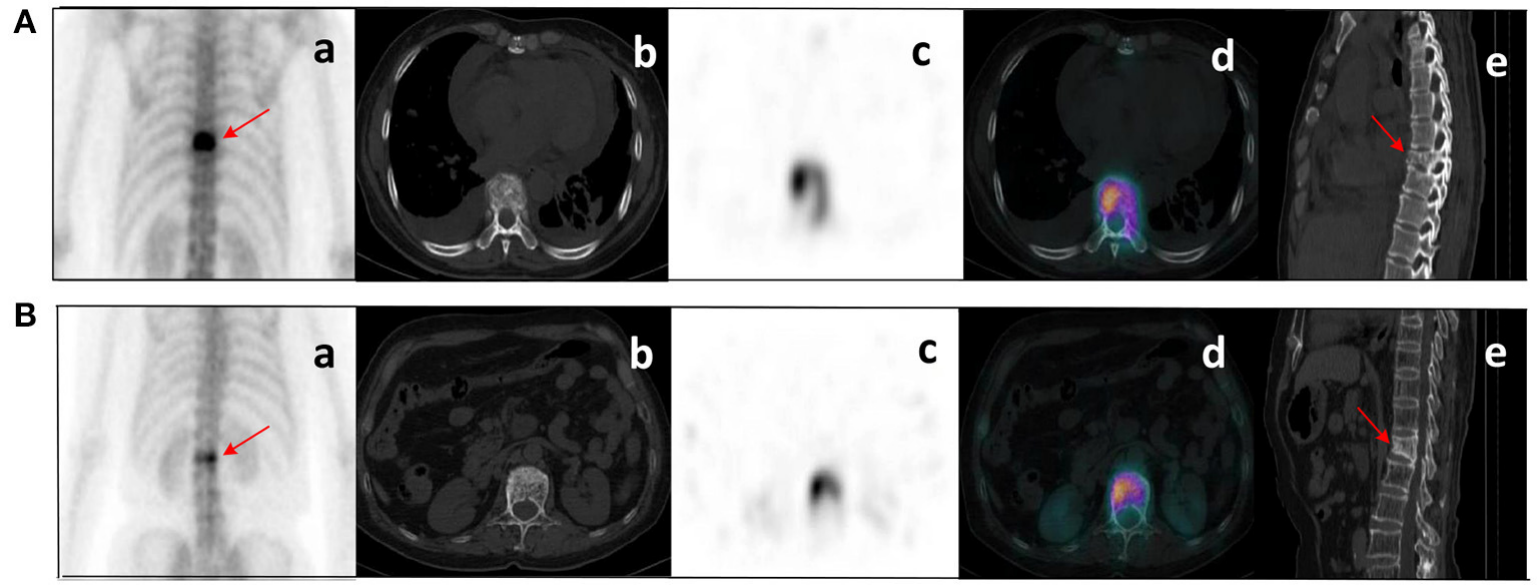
Clinical cases SPECT/CT images of bone metastases (A) and benign bone lesions (B). The images shown are WBS image, axial CT, SPECT, fusion image, and sagittal CT (a–e, respectively). (A) bone metastases: a 53-year-old female with an adenocarcinoma of the left lung. Wedge-like changes of the T8 vertebral body with an abnormal concentration of radioactive tracer (arrows). (B) benign bone lesions: a 68-year-old female with breast cancer. Wedge-like changes of the L1 vertebral body with higher bone density and increased radioactive tracer distribution (arrows). It was difficult to determine whether lesions were metastasis with conventional images only. Lesion (A) was confirmed as pathological fracture due to bone metastases by pathological examination and showed systemic bone metastases at subsequent imaging follow-up. Lesion (B) was confirmed to be a benign compression fracture by imaging follow-up and clinical information.
